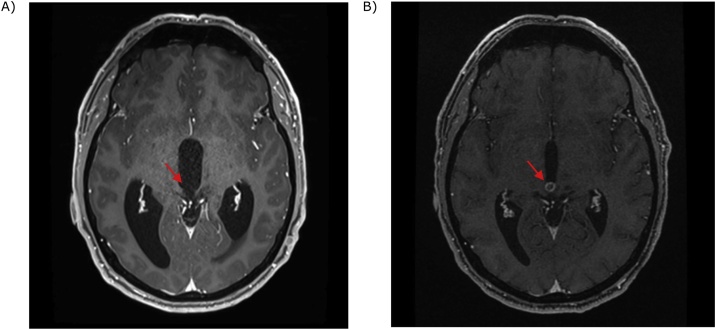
MRI brain showing: A – Pre-operative Axial T1 sequence with contrast, lesion is non-enhancing (arrow) and hydrocephalus is evident; B – same sequence at 5 months post-operation demonstrating rim enhancing lesion (arrow) and resolution of hydrocephalus.
